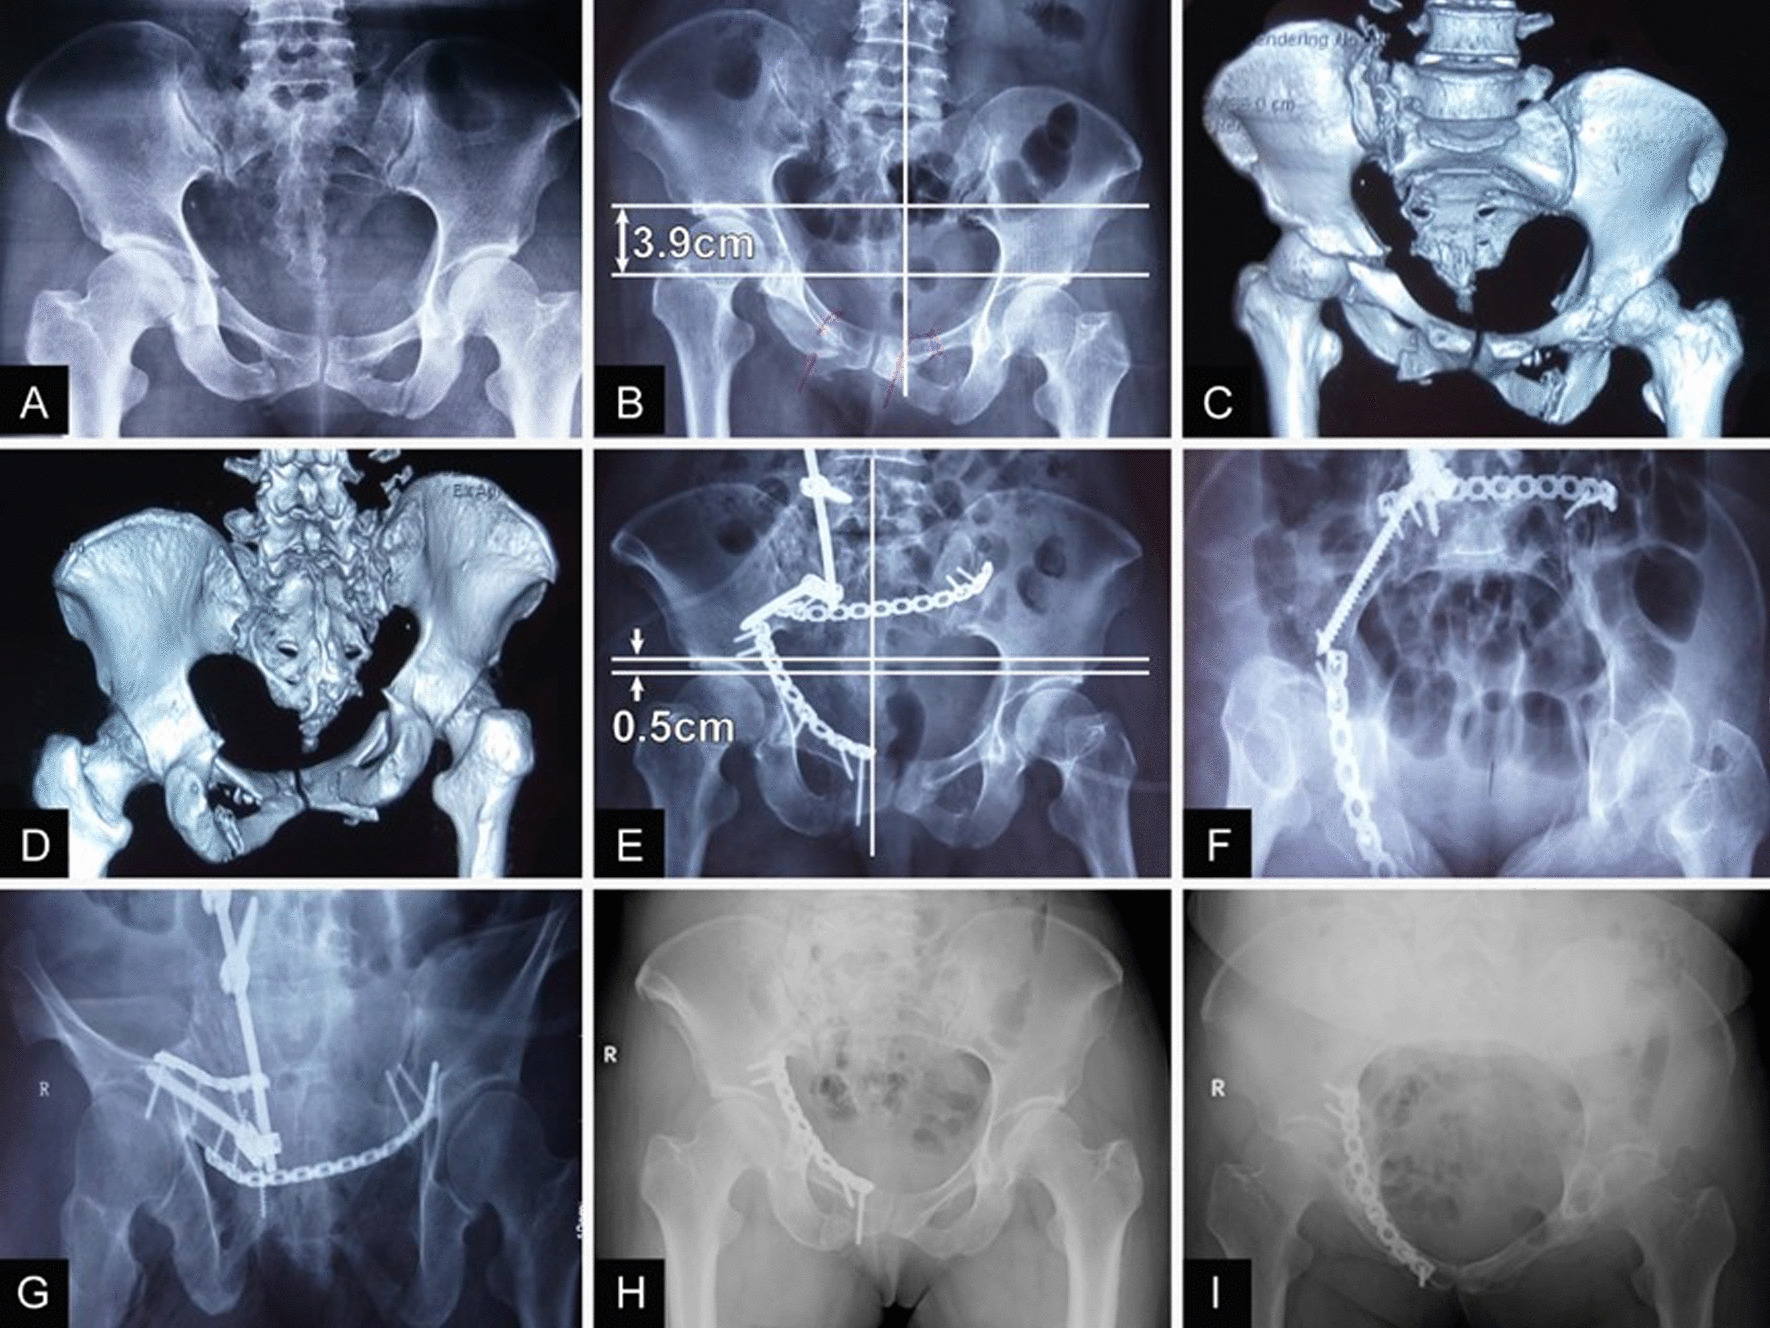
A 45-year-old female patient. a AP X-ray at the time of injury showing the pelvic ring without obvious cranial displacement. b AP X-ray showing 3.9 cm of unilateral cranial pelvic displacement after 1 month of conservative treatment. c, d Three-dimensional CT showing sacral malunion at 3 months after the failure of reduction of femoral skeletal traction. e Postoperative AP X-ray showing a residual displacement of 0.5 cm after reduction. f, g Inlet and outlet X-ray images showing reduced hemipelvic displacement. h, i Pelvic radiographs acquired 15 months after the operation showing healing of the fracture and removal of the instrumentation for internal posterior pelvic ring fixation
